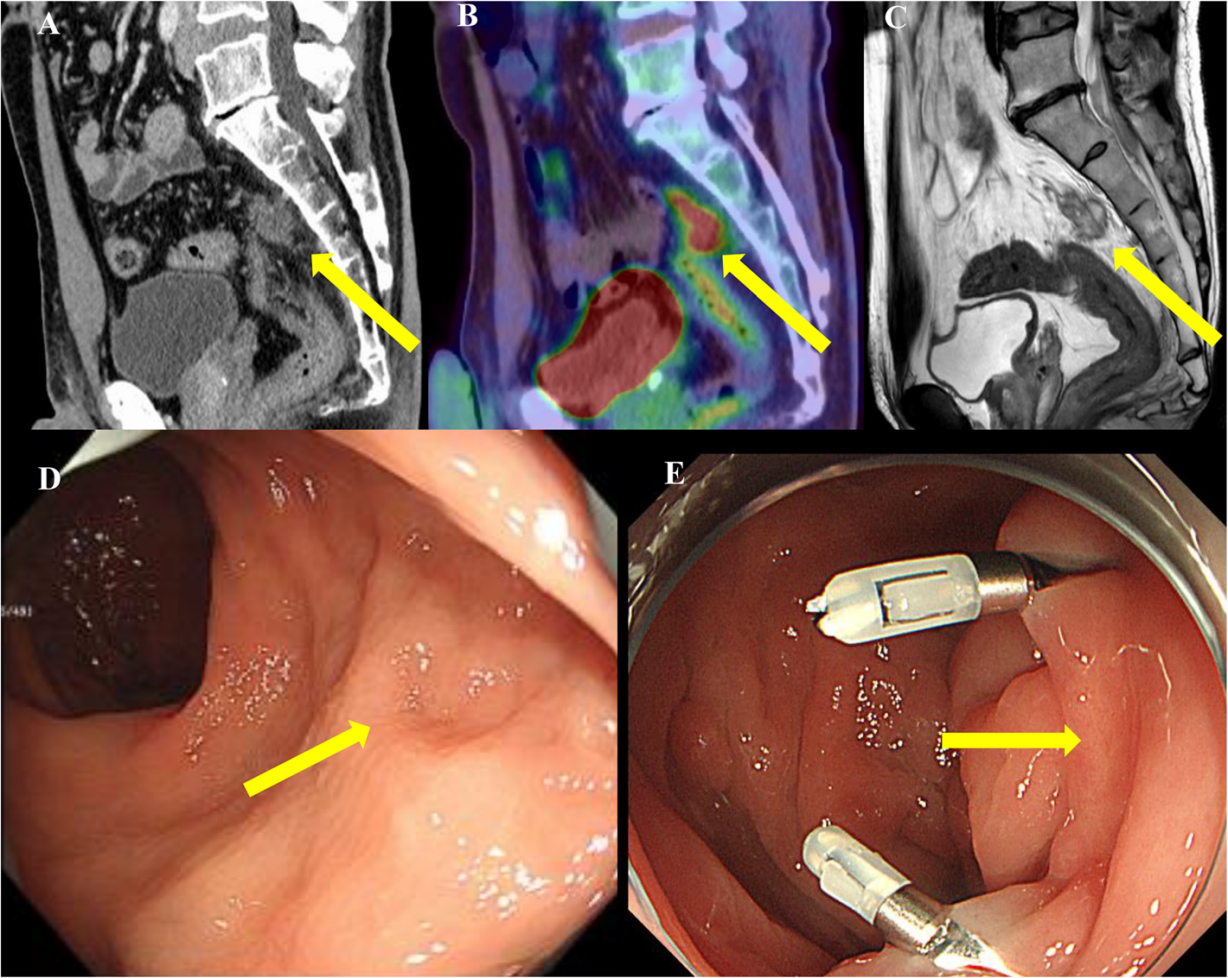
Preoperative imaging showing the recurrent tumor at the presacral area. a, b PET-CT shows a tumor with an abnormal FDG uptake at the presacral area. c MRI shows no evidence of invasion to the rectum or sacrum. d Endoscopy showed the EMR scar at the upper rectum, but no evidence of new mucosal lesions. e Clipping and marking near the scar were performed endoscopically
